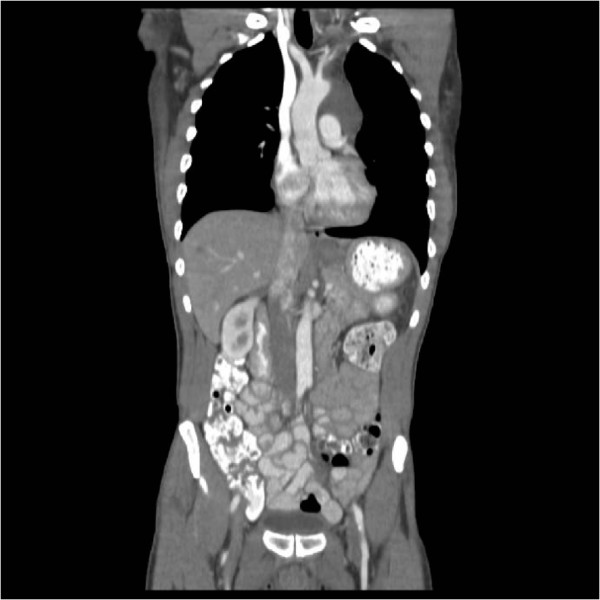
CT scan showing resolution of tumor at 3 months.
